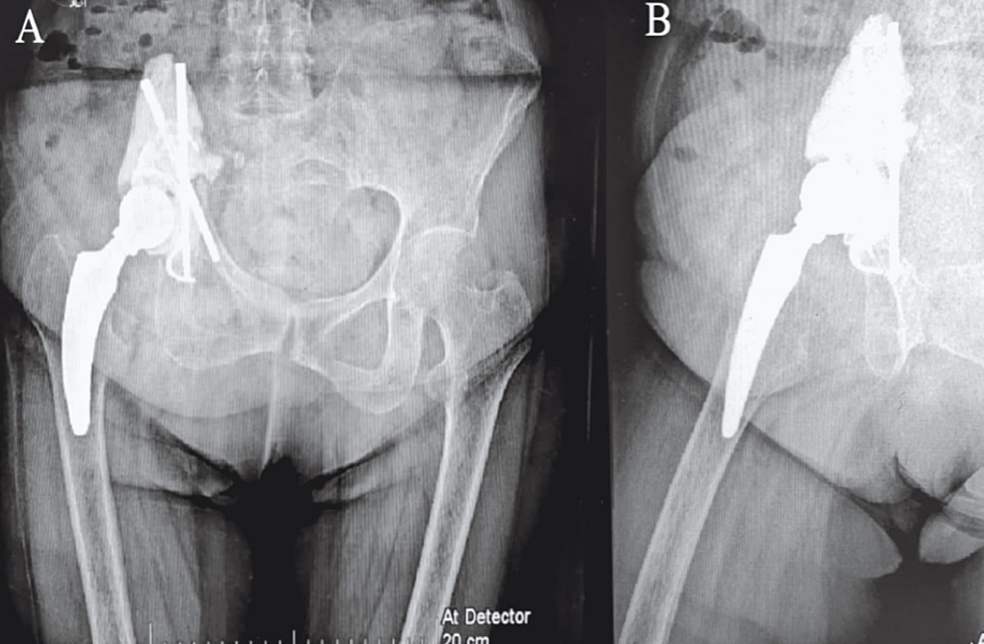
Postoperative X-ray at seven months of control. (A and B) Anteroposterior views in neutral and abduction showing a fixed prosthesis, with medialization and vertical final position of the cup.
